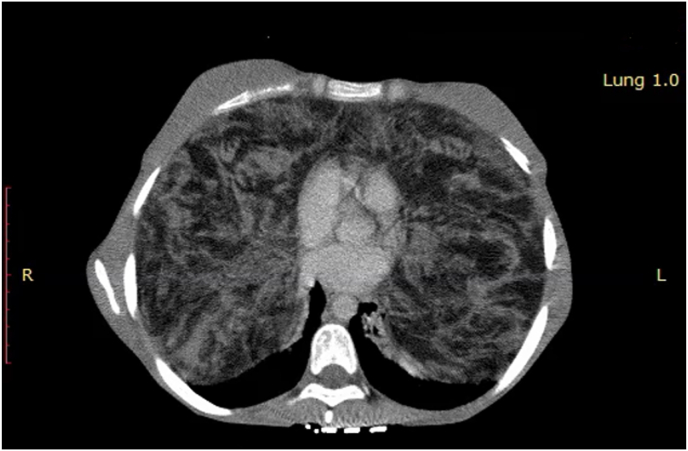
Patient's chest CT: a mass taking up most parts of both chest cavities and measuring 36 × 29 × 10 cm.
